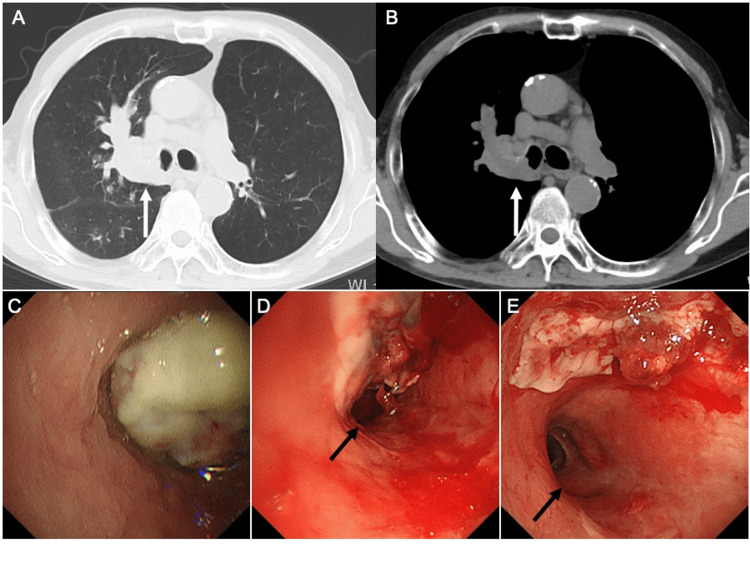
Chest computed tomography at admission shows the mass progressing to the right main bronchus, obstructing the bronchial lumen (white arrow) (A, B). Bronchoscopy before cryotherapy shows almost complete obstruction of the right main bronchus by a mass extending from the orifice of the right upper lobe bronchus (C), and following bronchoscopic cryotherapy, shows partial relief of the right main bronchus obstruction and patency of the truncus intermedius (black arrow) (D). Bronchoscopic image after repeat bronchoscopic cryotherapy, which was performed three days later, shows residual tumor protruding into the right main bronchus and patency of the truncus intermedius (black arrow) (E).
